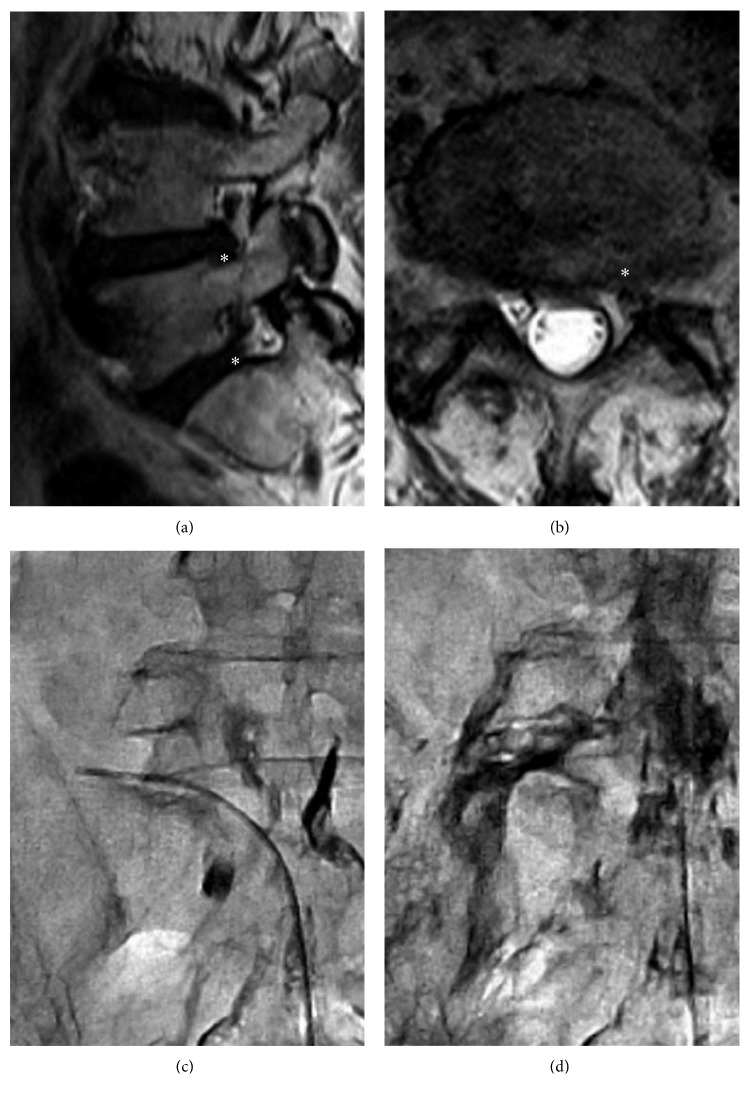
Representative case of a 60-year-old man with radicular pain in the left leg who underwent lumbar PEN. (a, b) Magnetic resonance imaging shows lumbar disc herniation and foraminal stenosis at the level of L4-5-S1 (asterisks). (c) The first epidurogram shows a filling defect at the left L5-S1 foramen. The catheter is inserted to the stenotic foramen, and mechanical adhesiolysis is attempted. (d) The final postadhesiolysis epidurogram shows excellent extraforaminal contrast spread. The patient exhibited significant pain relief and functional recovery after PEN. PEN: percutaneous epidural neuroplasty.
